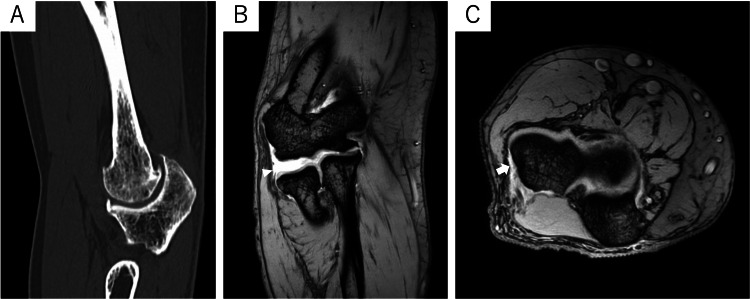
Preoperative CT and MRI scans of the right elbow of case two (A) CT scans showed osteoarthritic changes in the right ulnohumeral joint. (B) The coronal T2*-weighted MRI scan showed high-intensity signal enhancement in the lateral collateral ligament (LCL), indicative of a rupture (white arrowhead). (C) Axial T2*-weighted MRI showed high-intensity signal enhancement of the proximal attachment of the LCL (white arrow).
